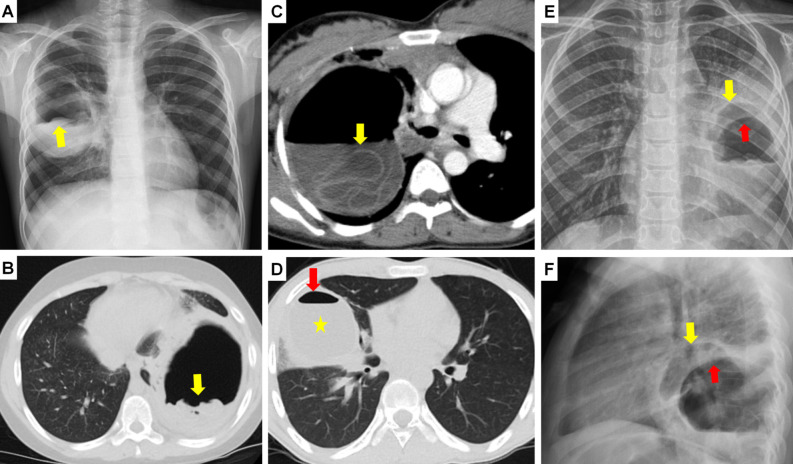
In different cases, chest radiograph (A) and axial computed tomography (CT) (B) show the “water lily or camolette sign” (arrow) formed by the collapsed endocyst floating on the cyst fluid. Axial CT (C) shows the “floating membrane sign” (arrow) formed by the endocyst becoming free after cyst rupture. Axial CT (D) shows the air-fluid level (arrow) formed after a portion of the cyst (asterisk) content emptied into the bronchus. Chest (E) and lateral radiograph (F) of different cases show the “cumbo or double dome arch sign” (arrows) formed by the observation of air-fluid levels within the endocyst and between the endocyst and pericyst, with the endocyst shrinking and rupturing due to increasing air amount.
